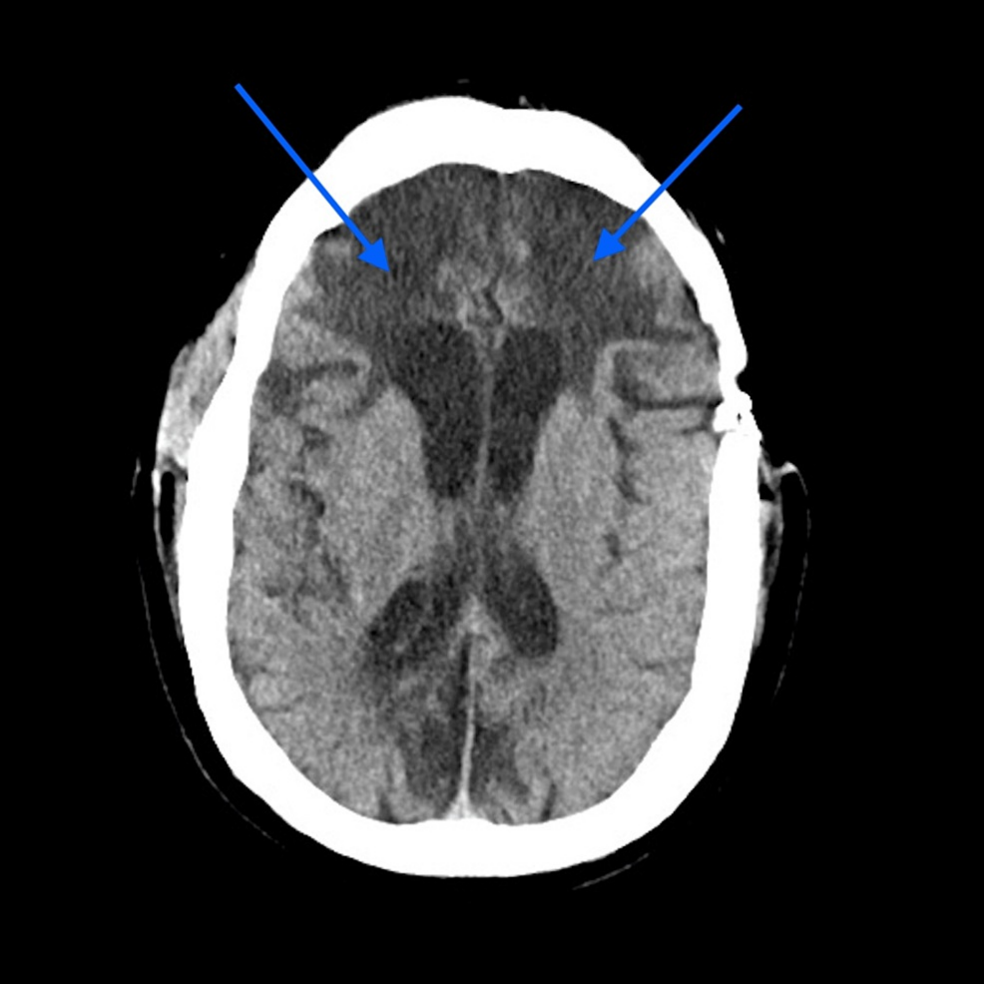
CT of the head - horizontal cut showing frontal lobe atrophy (blue arrows) CT: computed tomography
